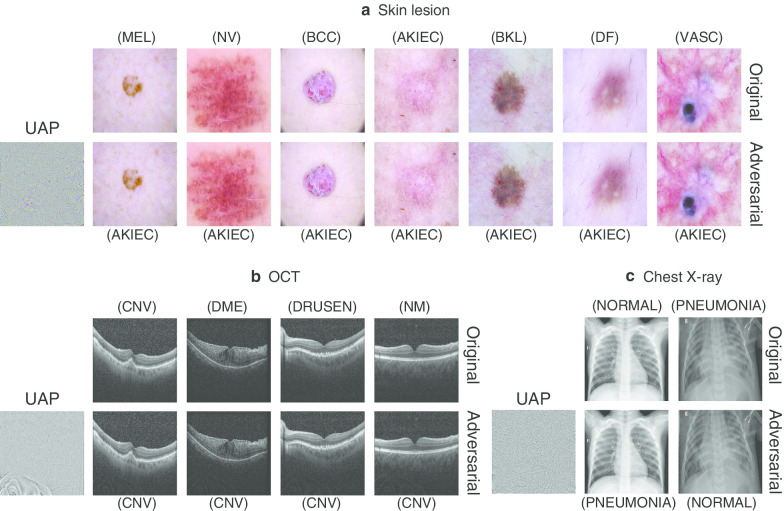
Nontargeted UAPs with $$p = 2$$ against Inception V3 models and their adversarial images for skin lesions (a), OCT (b), and chest X-ray image datasets (c). Further, $$\zeta = 4\%$$ in a and c. $$\zeta = 6\%$$ in b. Labels in brackets beside the images are the predicted classes. The original (clean) images are correctly classified into their actual labels. UAPs are emphatically displayed for clarity; in particular, each UAP is scaled by a maximum of 1 and minimum of 0
